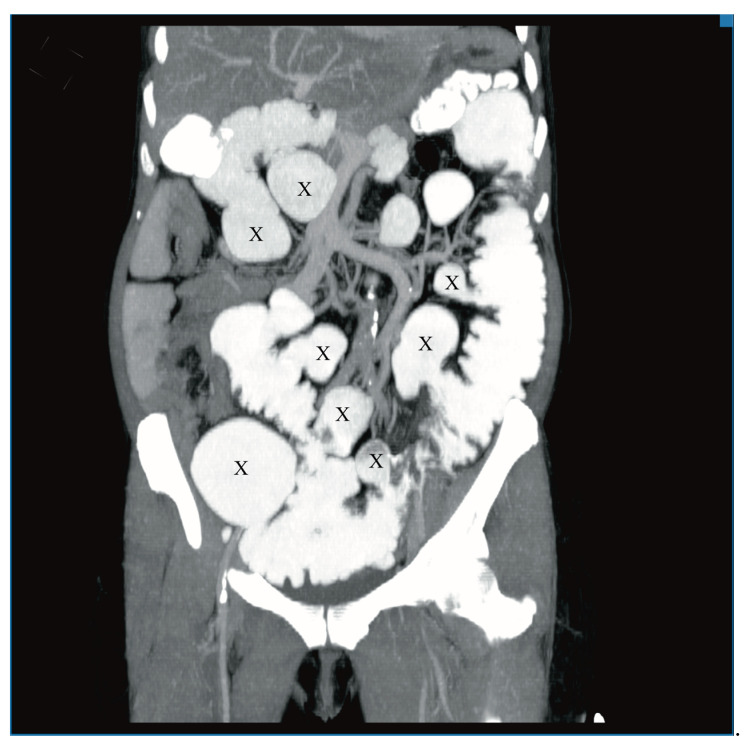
Contrast-enhanced CT image of the abdomen and pelvis, frontal section. Multiple giant diverticula (X).
